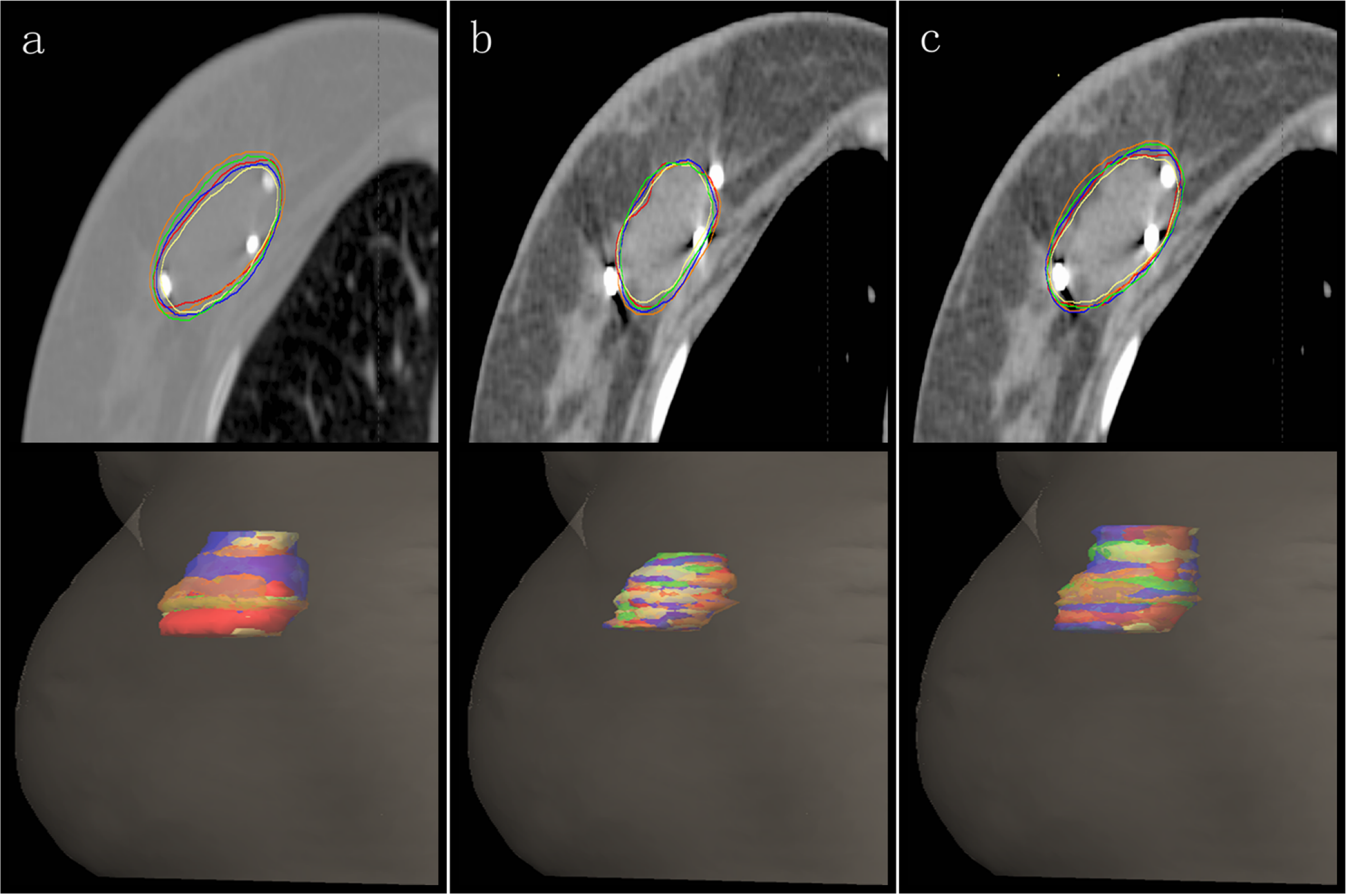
Single CT slice and volumetric image of one patient with delineated TB
